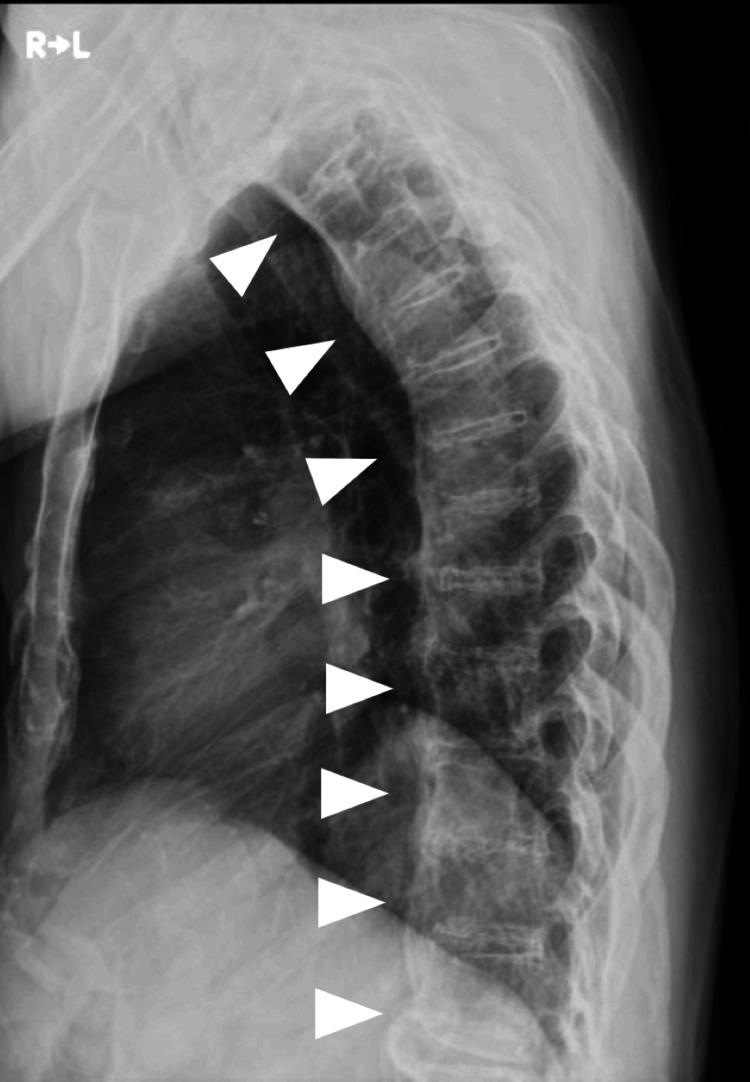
Plain X-ray of the thoracic spine Fusion of adjacent vertebral bodies is observed from the thoracic to lumbar spine due to ossification of the anterior longitudinal ligament (arrowhead).
